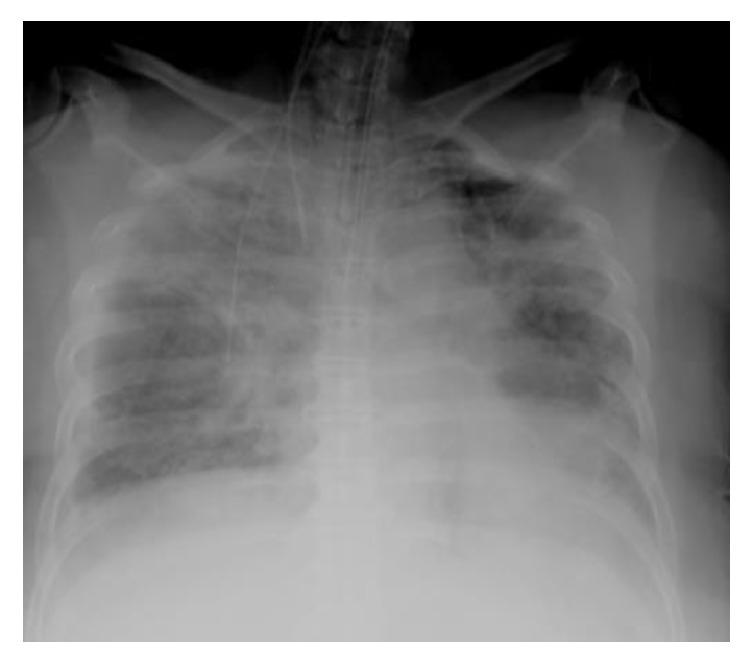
X-ray before transplant.
